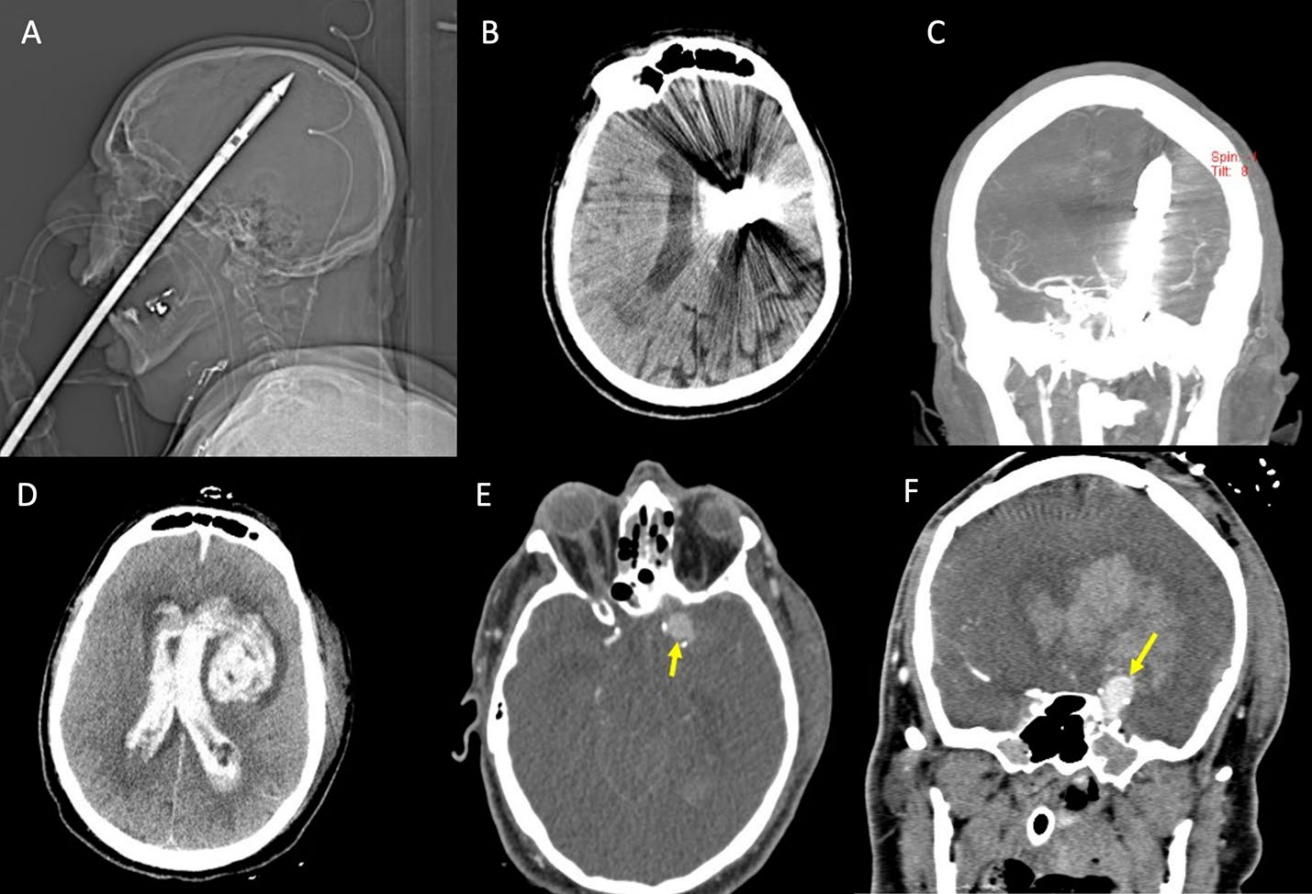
Patient in the sixth decade of life with a penetrating spear gun harpoon trauma (A, scout image). The harpoon penetrated the brain through the hard palate and the cranial base, with absence of significant cerebral hemorrhagic hyperdensity on non-enhanced CT (B), and a possible slight narrowing of the left distal middle cerebral artery on CTA (C), in the presence of metal artifact. Bottom row shows the post-surgical control, after removal of the harpoon, demonstrating the occurrence of ventricular hemorrhage and left intraparenchymal hematoma on non-enhanced CT (D), with evidence of a pseudoaneurysm arising from the supraclinoid left internal carotid artery on CTA (E and F, arrows)
